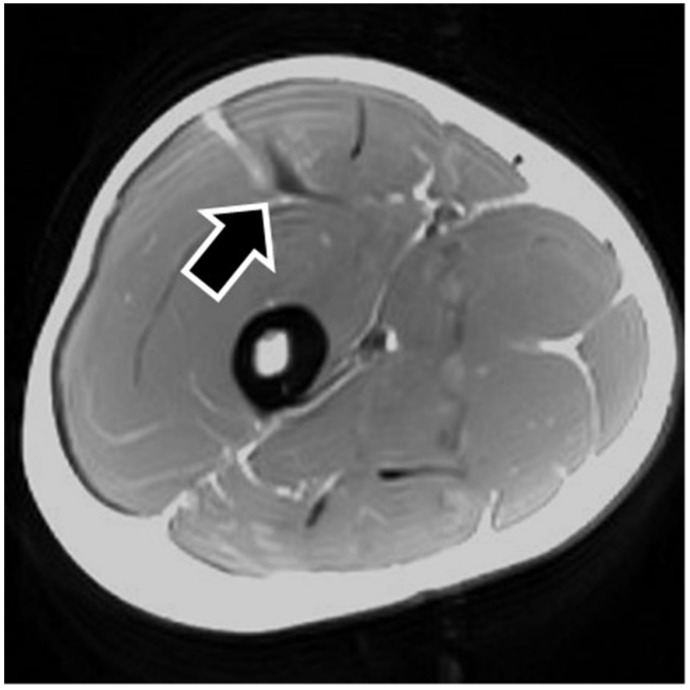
Injured rectus femoris muscle with a history of affecting the myofascial junction. The figure shows an example of myofascial junction injury. The axial T1-weighted image shows a low signal in the myofascial junction of the rectus femoris muscle (black arrow).
